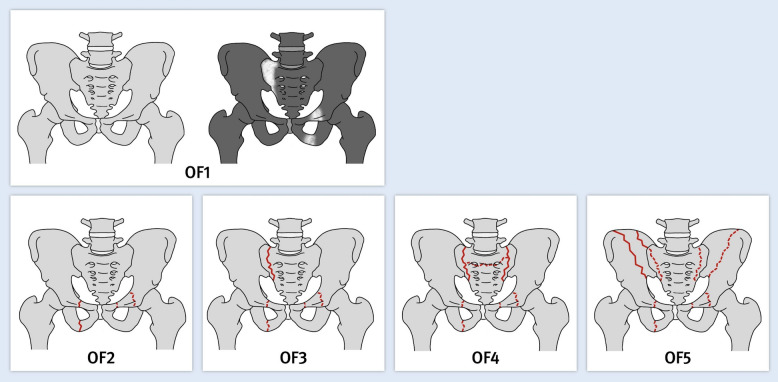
The OFP-Classification is presented: OF1: edema in the pelvic ring at one or more locations (no evidence of fractures on CT); OF2: anterior fracture of the pelvic ring on one or both sides; OF3: unilateral sacral fracture with or without fracture of the anterior pelvic ring; OF4: bilateral sacral fractures with or without fracture of the anterior pelvic ring including H- or U-type fractures; OF5: iliac or transiliosacral fracture with or without fracture of the anterior pelvic ring. Solid lines are variants for the fractures of the different OFP fracture types: dashed lines are optional additional fracture localizations. MRI or dual energy CT is required for edema detection
